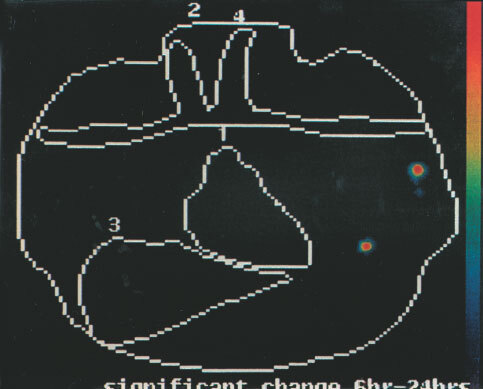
Patient CP 4, Tc-99m hHMFG-1 radioimmunoscintigraphy. Positive left axillary nodes and breast cancer.
